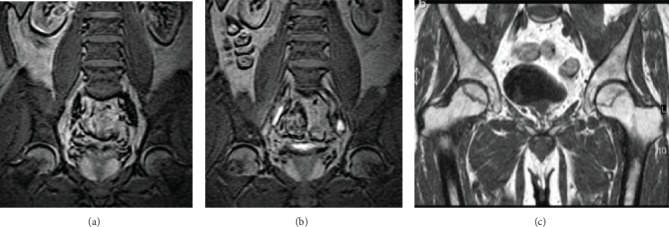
A 64-year-old male developed ONFH after DBRs treatment for 1.6 months. (a, b) Coronal magnetic resonance image before DBRs. (c) Coronal magnetic resonance image after cumulative dose of 200 mg dexamethasone for 1.6 months. ONFH: osteonecrosis of femoral head; DBRs: dexamethasone-based regimens.
